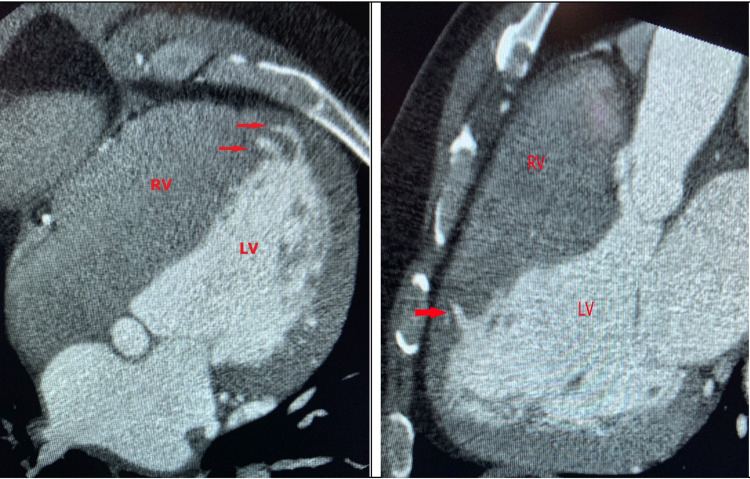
Patient #16’s CTA images of the clefts (red arrows) and ventricular structures (labeled in red); the clefts are extending from the anterior septum to the inferior septum CTA, Coronary computed tomography.
